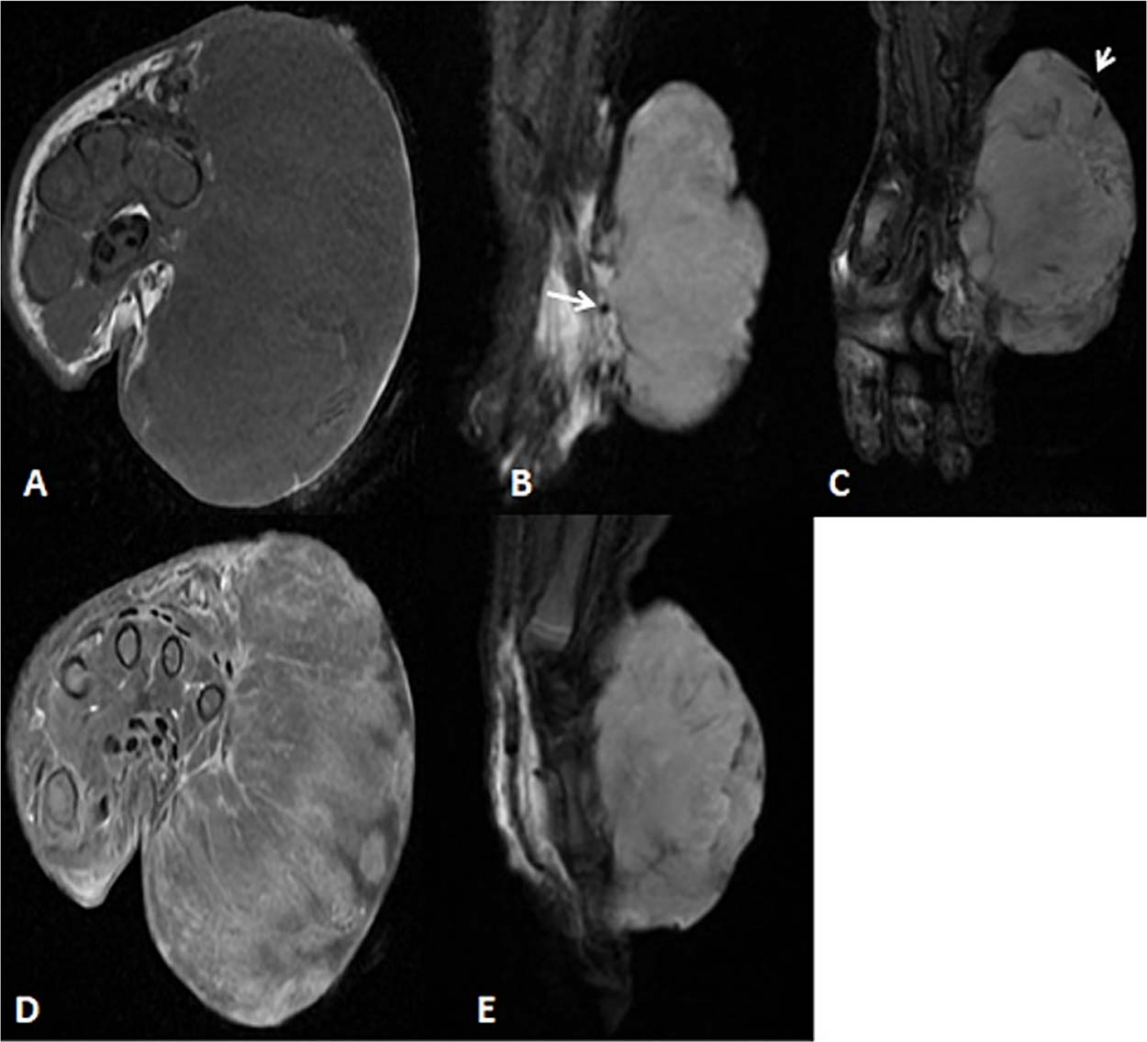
MRI of left hand mass. (A) Axial T1WI shows a huge homogenous isointense mass to the corresponding hand muscles signal intensity, which is seen along the medial aspect of left hand. (B and C) Coronal STIR demonstrate heterogeneous diffuse high signal intensity of the lesion and appears inseparable with sign of invasion to the fourth and fifth digits extensor compartment/tendons as well as presence of areas of signal void (arrow). (D and E) Axial and sagittal post-contrast *T*_1_ fat-saturated images show diffuse progressive intense enhancement of the lesion.
